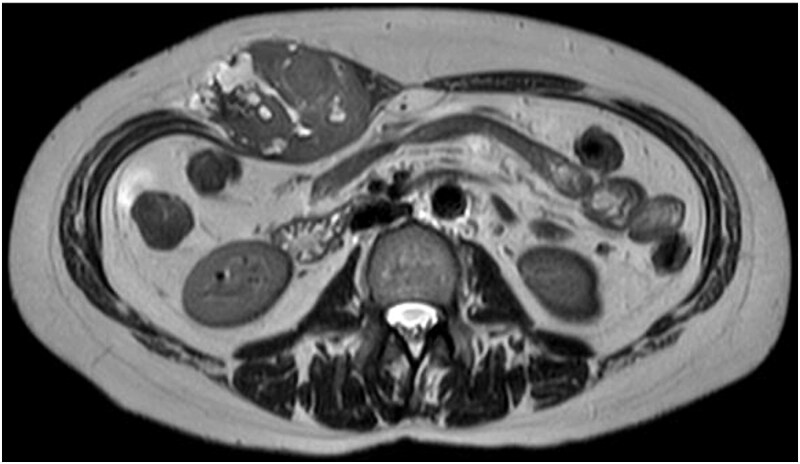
T2WI MR image showing an expanding mass after denosumab administration.
